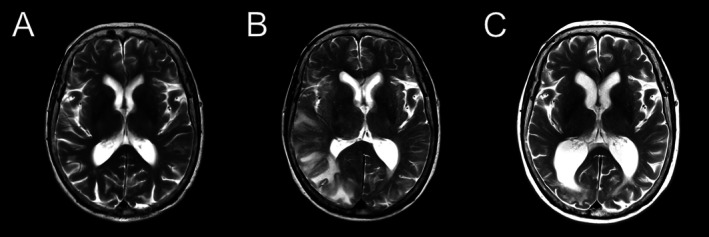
Brain volume loss after cerebral amyloid angiopathy‐related inflammation on serial T2 magnetic resonance imaging. (A) T2 images of the same patient showed a brain image 1 year before the onset of inflammation; (B) the image at the time of active inflammation; and (C) the follow‐up image 7 months later, showing volume loss with *ex vacuo* dilatation of the trigone of the right lateral ventricle.
